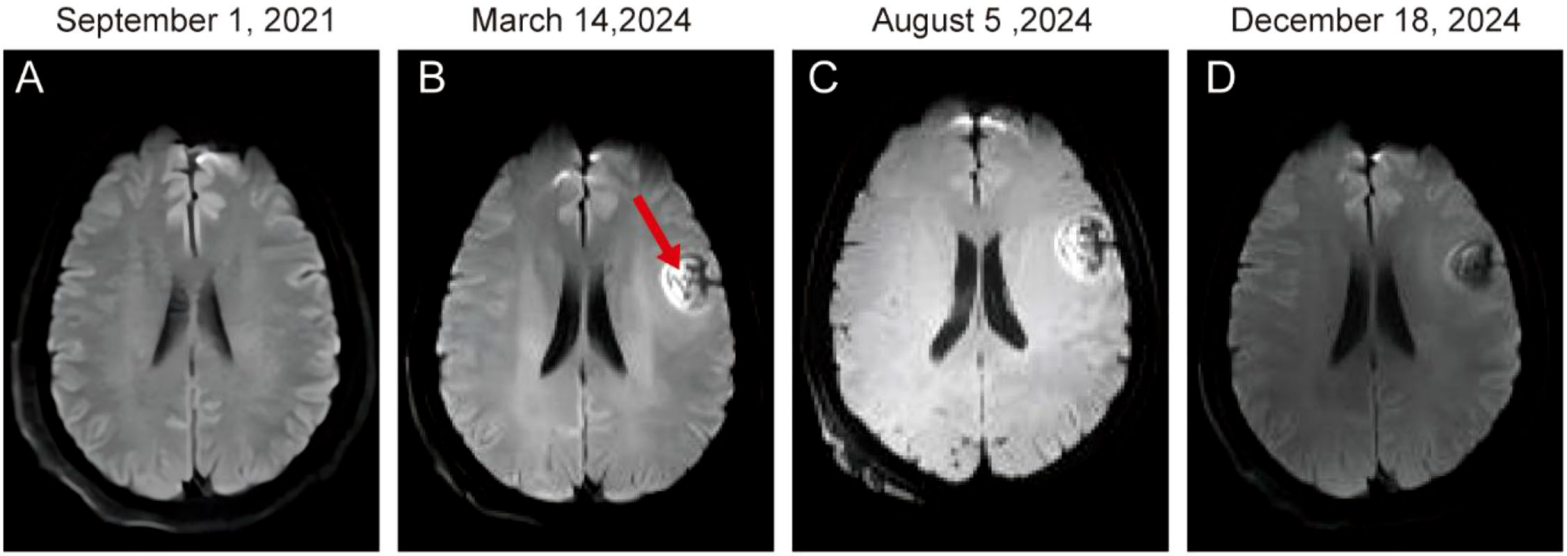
Magnetic resonance imaging (MRI)of a 54-year-old female with recurrent malignant melanotic nerve sheath tumor with brain metastases. Diffusion-weighted image (DWI) shows mixed signal intensity of the right frontal (A-D).
